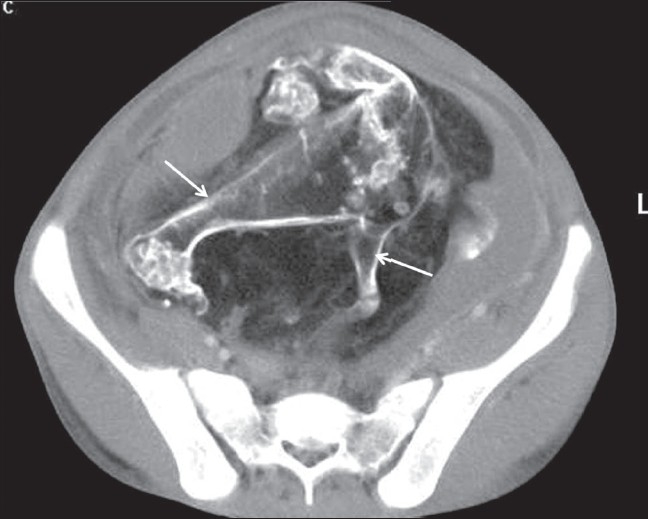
Axial contrast-enhanced CT scan shows ossified extremities (arrows) of the bigger fetus
